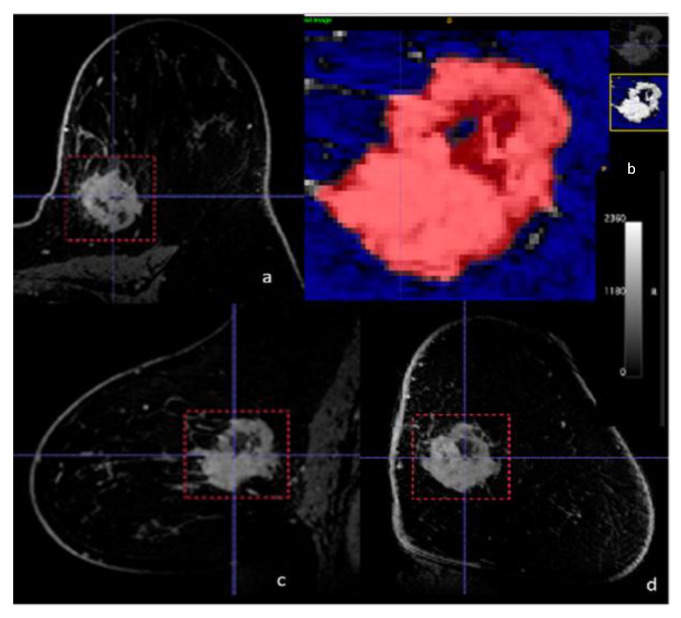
Example of semi-automatic tumor segmentation with dynamic contrast-enhanced MRI—(a) axial, (b) axial detail, (c) sagittal, (d) coronal—for radiomics analysis in a 43-year-old female with a biopsy-proven PD-L1-positive poorly differentiated triple negative breast cancer in the 10:00 axis of the left breast.
